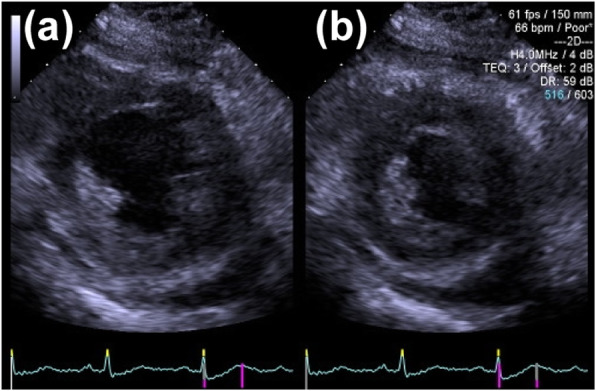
Echocardiography at short axis view. a Diastole b Systole. Hypokinesis of the inferior wall motion and a moderate amount of pericardial effusion are noted
